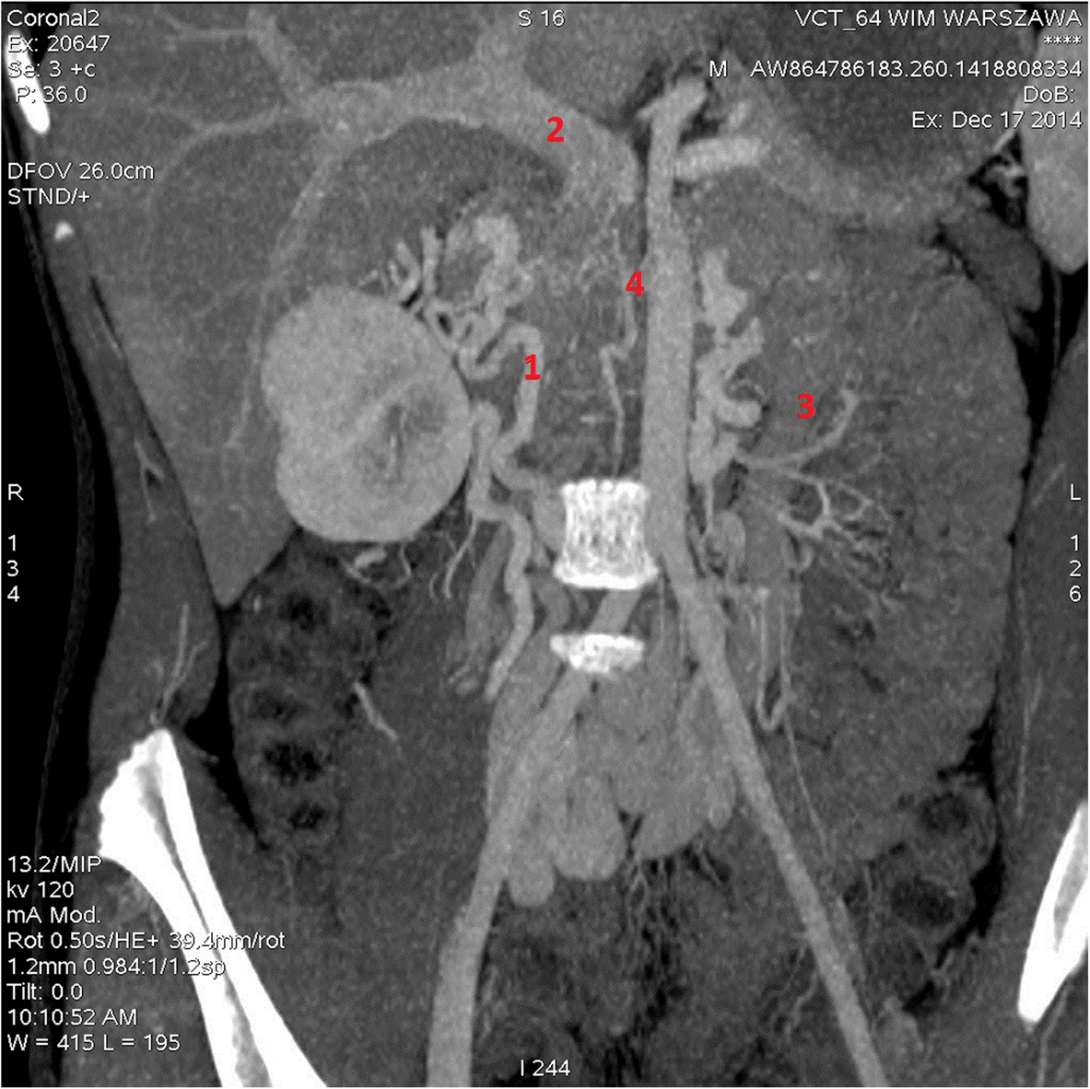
CT-angiograph of 14-year old boy with absence of inferior vena cava. Scan shows lack of contrast filling at the site of vena cava inferior. Numerous veins of collateral circulation within pelvis are varicosely dilated. Because of their atypical anatomy drainage of the renal veins could not be identified.1. Renal confluence, 2. Dilated portal vein, 3. Inferior mesenteric vein, 4. Lack of contrast filling at the site of inferior vena cava.
